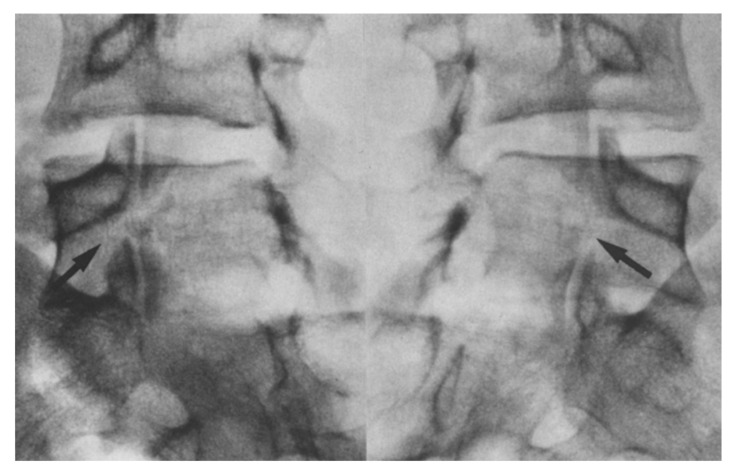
Oblique radiographs showing a bilateral pars defect in the L5 of a 17 year old patient [45]. The arrows depict the specific sites of the defect. “Used with permission of Springer Nature BV, from Hefti, F.; Seelig, W.; Morscher, E. Repair of lumbar spondylolysis with a hook-screw. *Int. Orthop.* 1992, *16*, 81–85; permission conveyed through Copyright Clearance Center, Inc.”.
